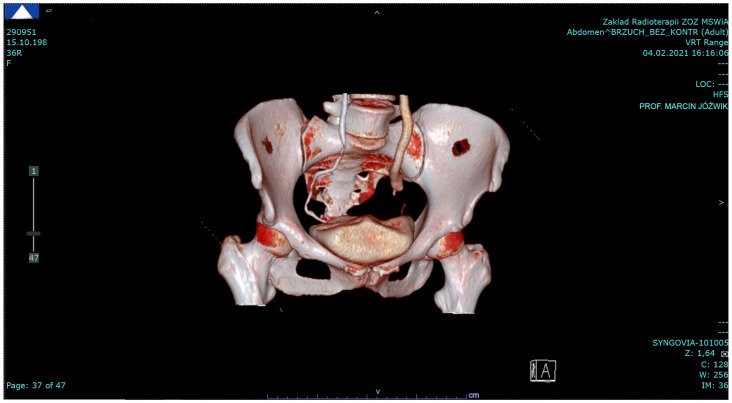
A preoperative CT scan demonstrating the normal right ureter and the left ureter dilated above its stricture at the ovarian fossa level (4 February 2021).
